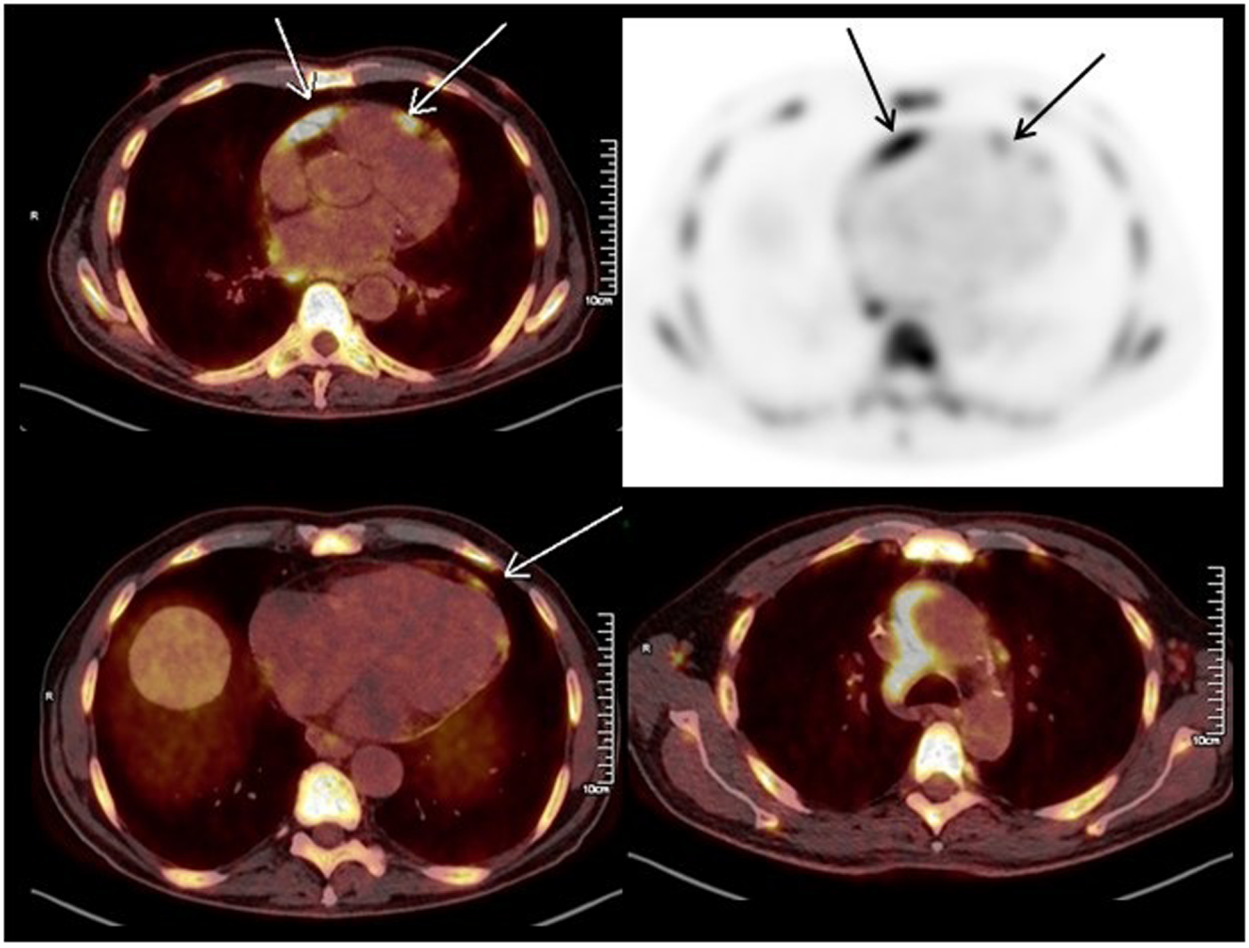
Positron emission tomography-computed tomography performed before CAR-T cell therapy showing multiple lymphoma lesions including in the pericardium (arrows).
